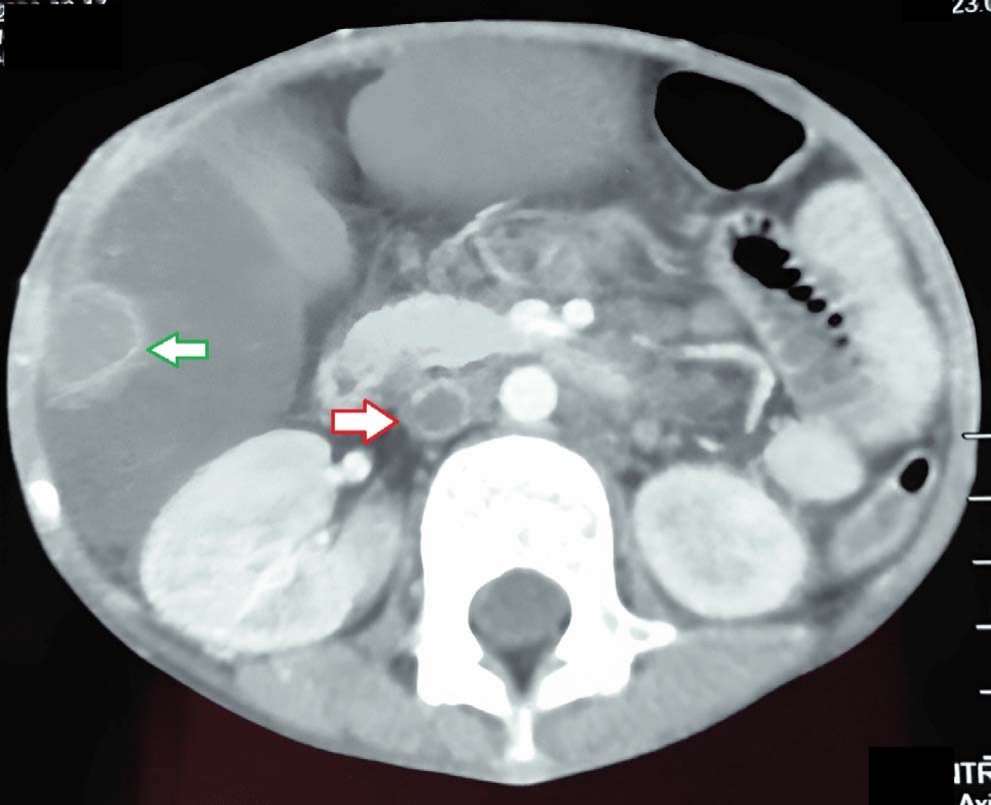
Contrast-enhanced computed tomography of the abdomen showed thrombus of the inferior vena cava starting at the level of the renal vein and extending toward the common iliac and external iliac vein on the right side (red arrow) and a hypoechoic mass in the liver (green arrow).
